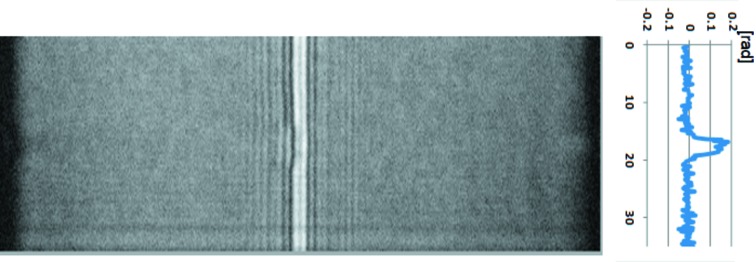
Raw image data of the SIXM-CT system obtained when the line-focused beam is located at the line *AB* shown in Fig. 5(*a*) ▶. Vertical line profiles of differential phase shift ∂*f*/∂*x* derived from the raw data by using equation (5)[Disp-formula fd5] are also indicated on the right-hand side. Exposure time is 60 ms.
